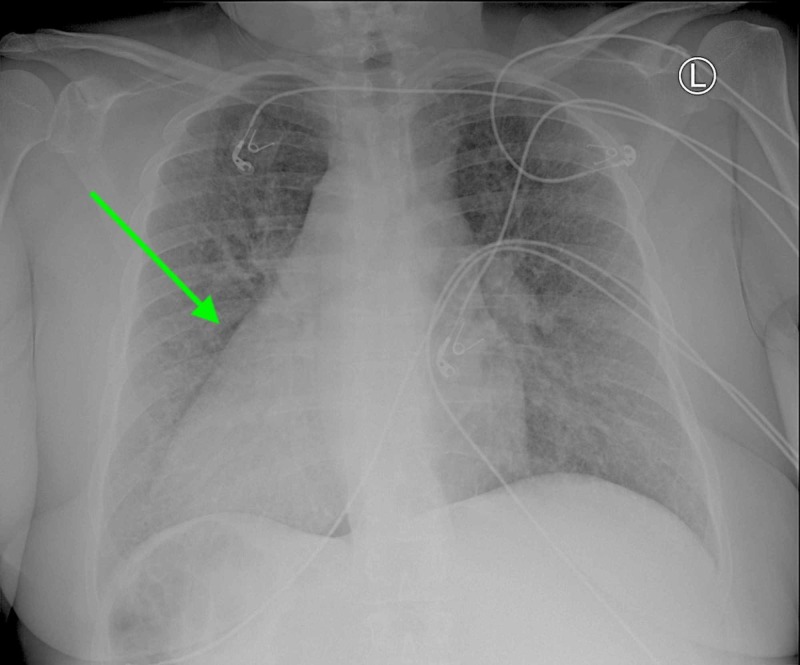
Anterior-Posterior chest X-ray demonstrating dextrocardia.
